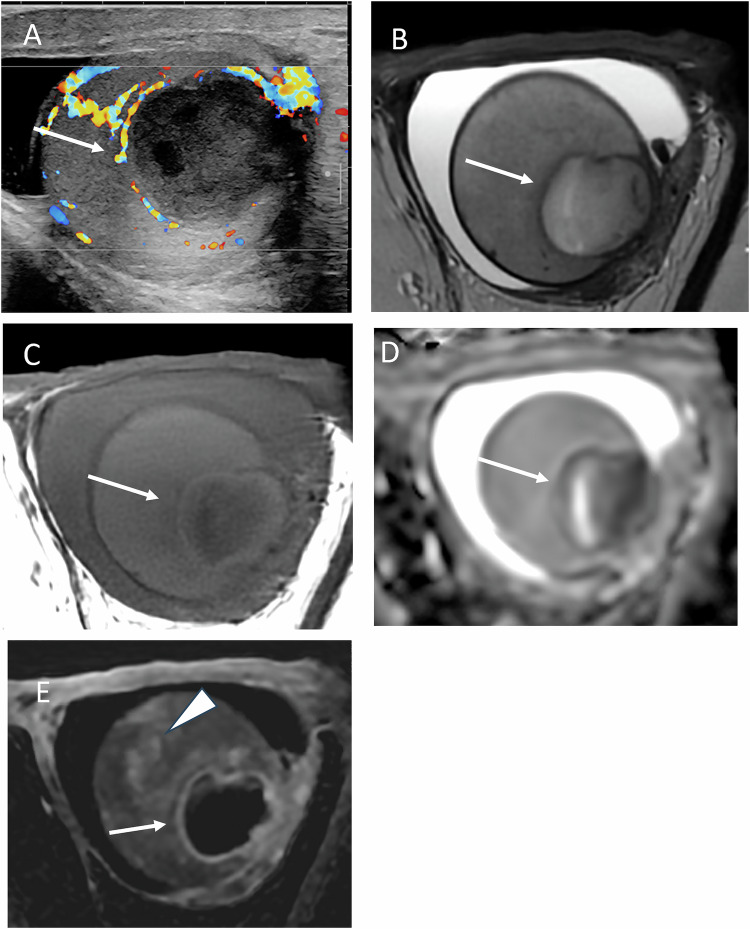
Syphilitic gumma in a patient with confirmed tertiary syphilis. Regression after appropriate antibiotherapy. A B-mode US coupled with Colour Doppler showing a heterogeneous lesion and absence of vascularity. B T2-weighted MRI showing mixed hyperintense and isointense signal. C T1-weighted MRI showing a very thin rim without clear hyperintensity. D ADC map showing heterogeneous signal. E Post-contrast T1-weighted MRI showing thin rim enhancement (long arrow) with small enhanced foci (arrowhead) and no internal enhancement
